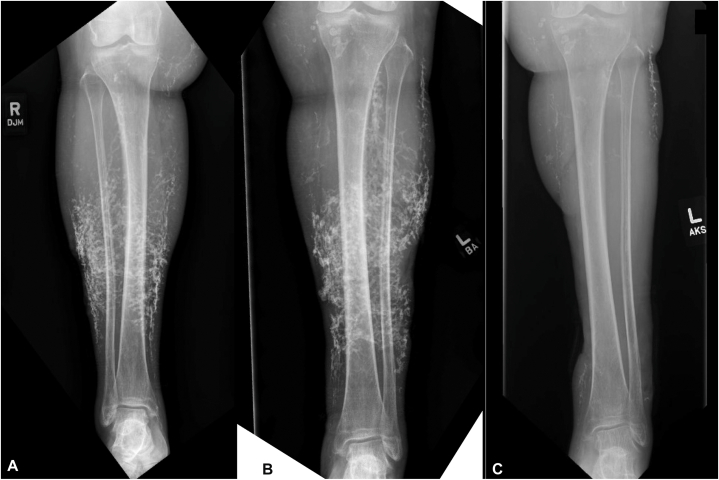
Radiographs of the right and left lower leg before surgery (A and B) and 17 months after surgery showing no calcinosis in the grafted areas on the left (C). Post-operative right-leg radiographs were not obtained.
